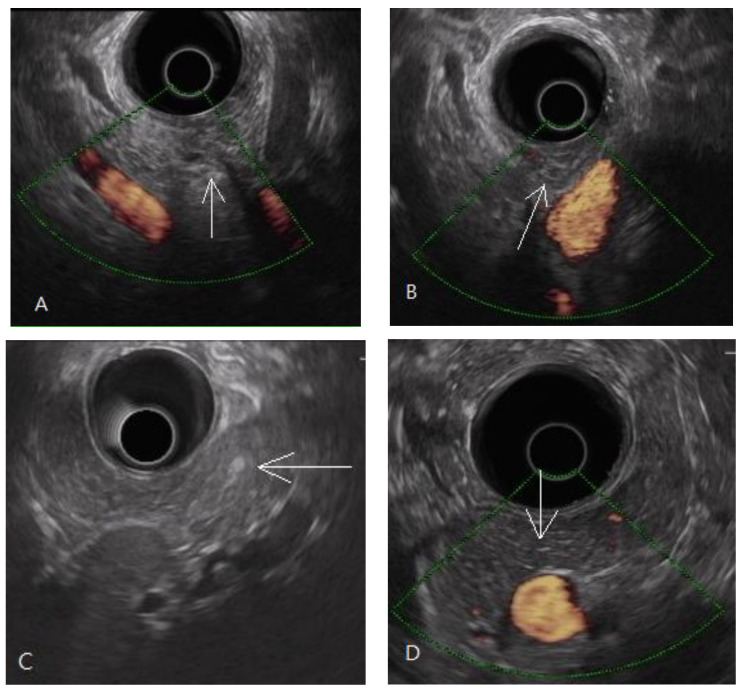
Endoscopic ultrasonography of early chronic pancreatitis. (A,B) Pancreatic head lobularity (arrow mark) showing heterogeneous parenchyma with hyper/hypo echoic areas mixing, irregular hyperechoic stranding. (C) A hyperechoic focus without an acoustic shadow (arrow mark) in the pancreatic tail. (D) Hyperechoic stranding in body, neck (arrow mark).
